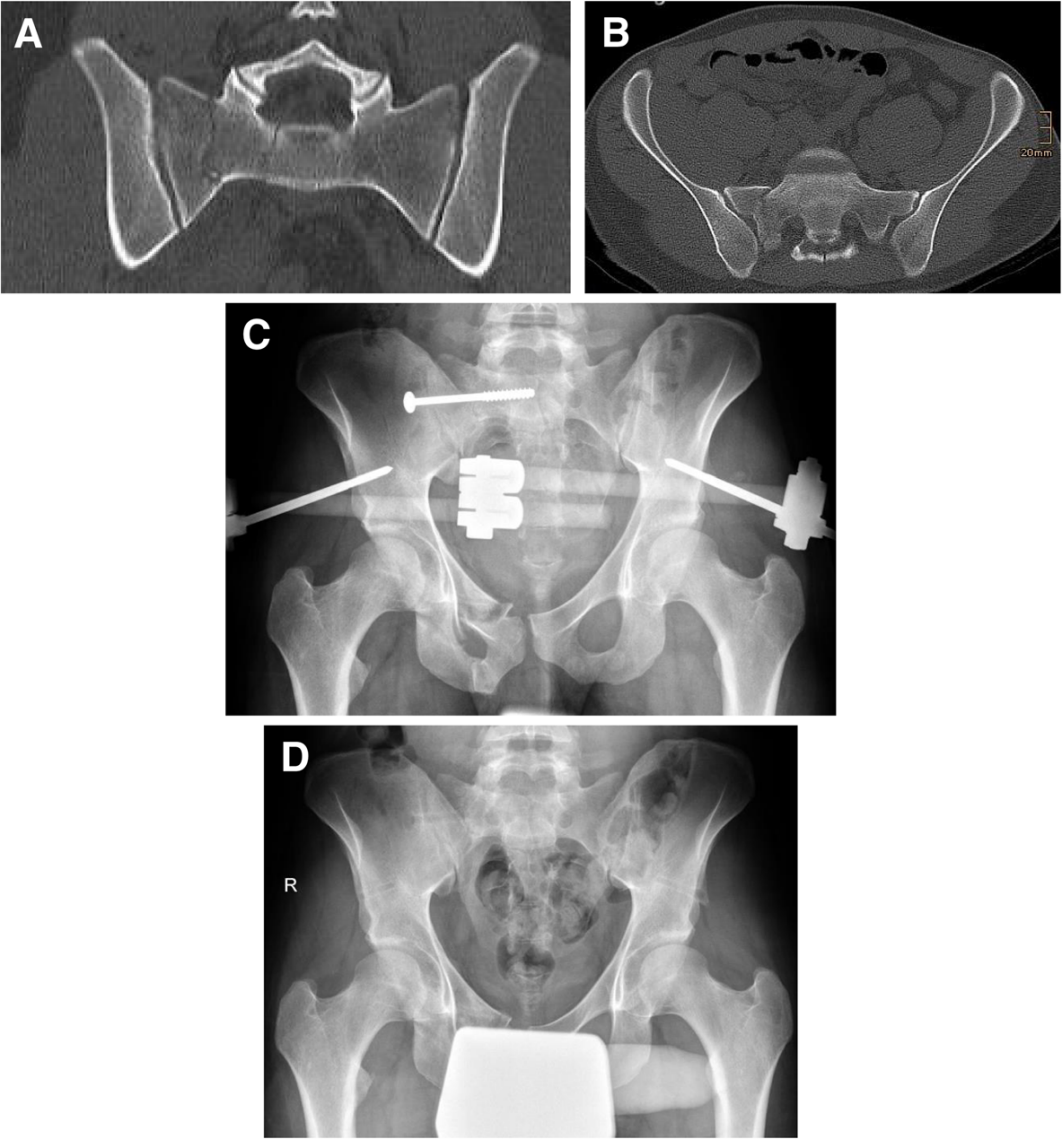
Clinical example for operative therapy of a lateral compression fracture. a and b show the initial CT-scanning with a sacral fracture on the right side. c shows the fixed situation after implantation of an ilio-sacral screw and anterior external fixation. d shows consolidation after implant removal 6 months following injury. The patient presented with full weight bearing and without pain
